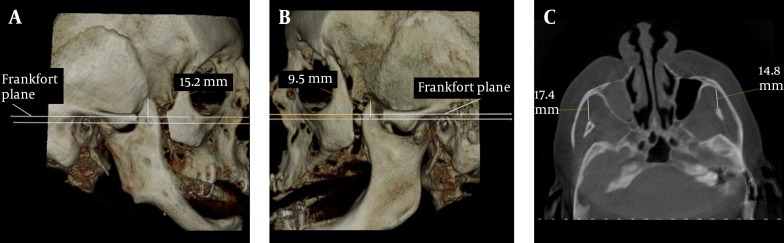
Case 2; A, 3D view of the elongated right coronoid process; B, 3D view of the elongated left coronoid process; C, The distance between the coronoid process and the inner face of the frontal part of the zygomatic bone on axial CBCT scan
